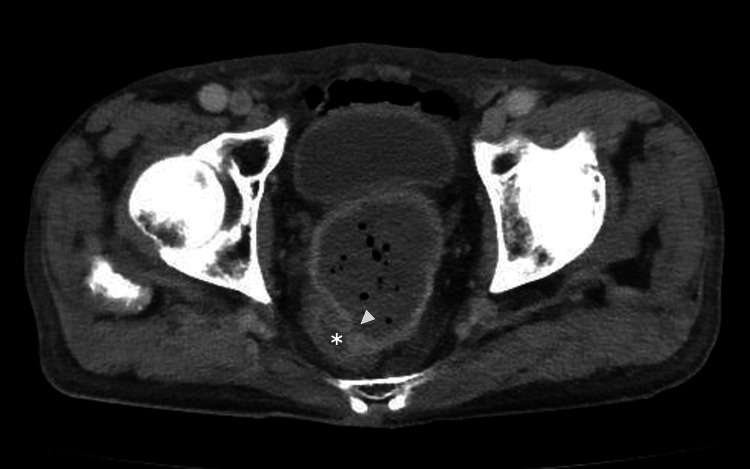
Contrast-enhanced CT indicates a rectal fistula caused by a left internal iliac artery aneurysm, characterized by ectopic gas within a giant aneurysmal sac and fistula formation (arrowhead) in the rectum (asterisk)
